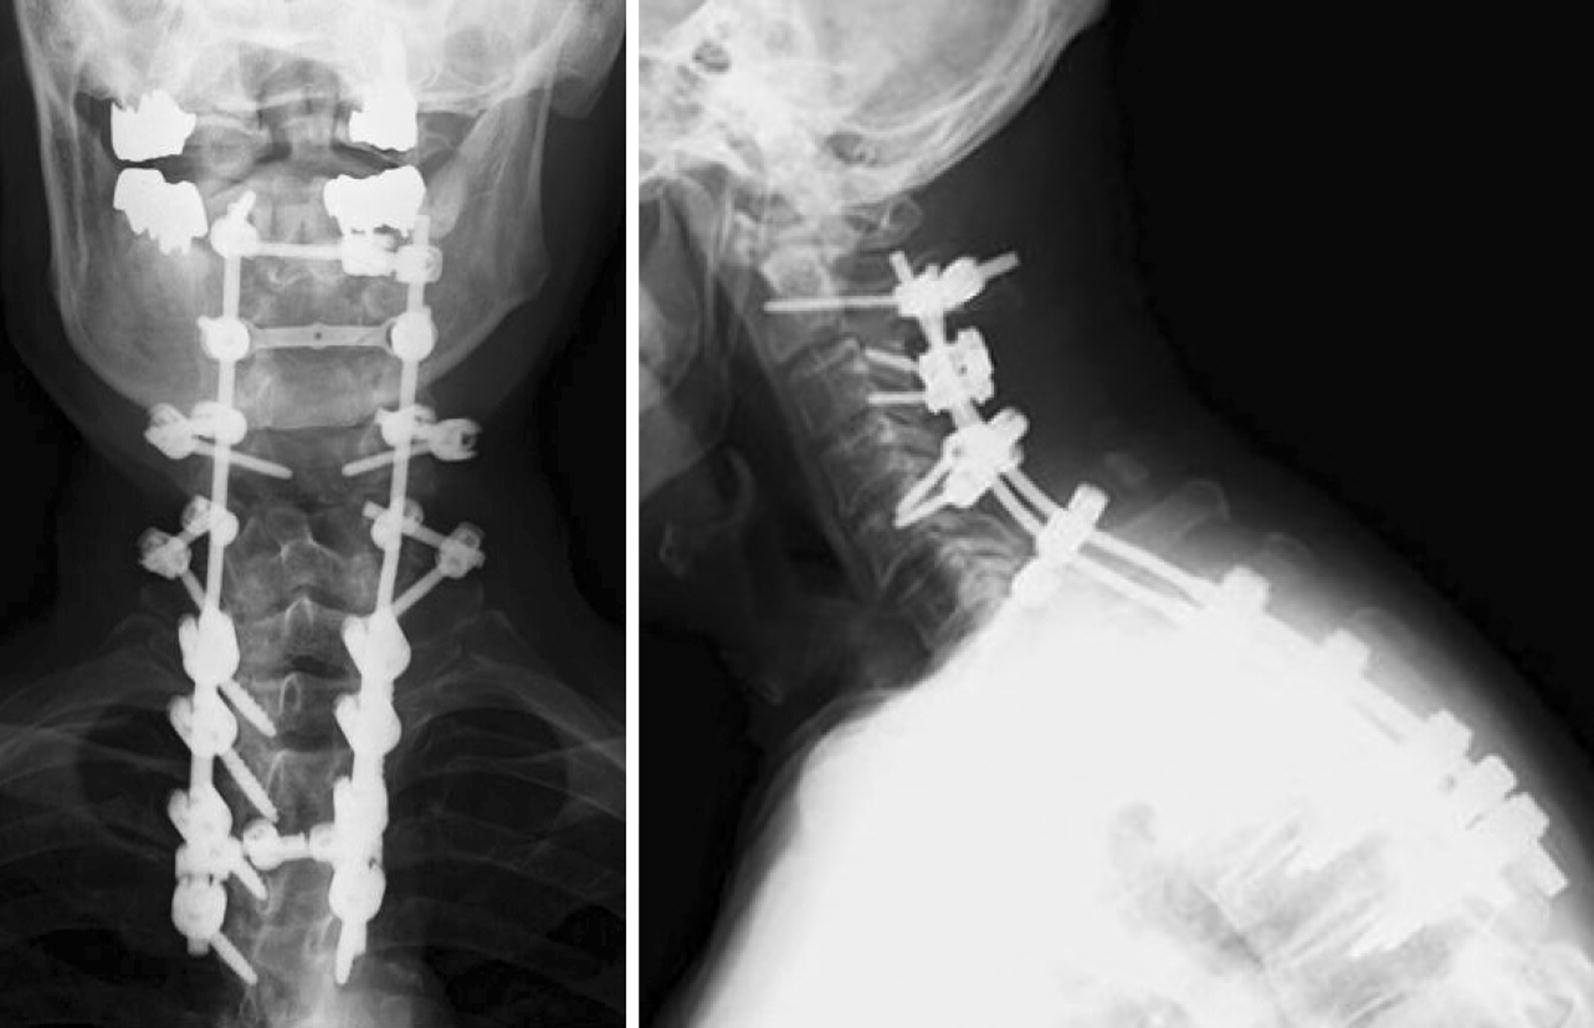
X-ray image at final follow-up
